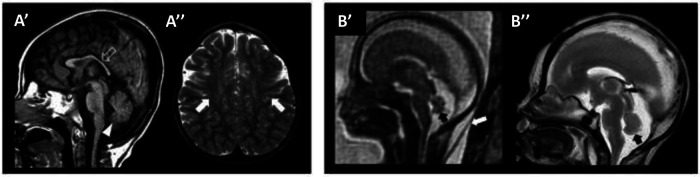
WOREE-associated neuroradiological features. A brain MRI of an affected patient was performed at 2.4 years of age (A’–A’’). (A’) The sagittal T1-weighted image reveals hypoplasia of the corpus callosum (empty arrow) and inferior cerebellar vermis (arrowhead). (A”) Axial T2-weighted images demonstrate mild atrophy of the frontal lobes with associated bilateral white matter hyperintensity (arrows). Fetal MRI (B’) and high-resolution post-mortem MRI (B”) of an affected patient were performed at 21 gestational weeks, demonstrating mild hypoplasia of the cerebellar vermis (black arrows). Note the slightly increased thickness of nuchal subcutaneous tissues on fetal MRI (white arrow). The laminar organization of the cerebral hemispheres and cortical gyration are appropriate for the gestational age (not shown). *Courtesy of Prof. Salpietro* (50).
